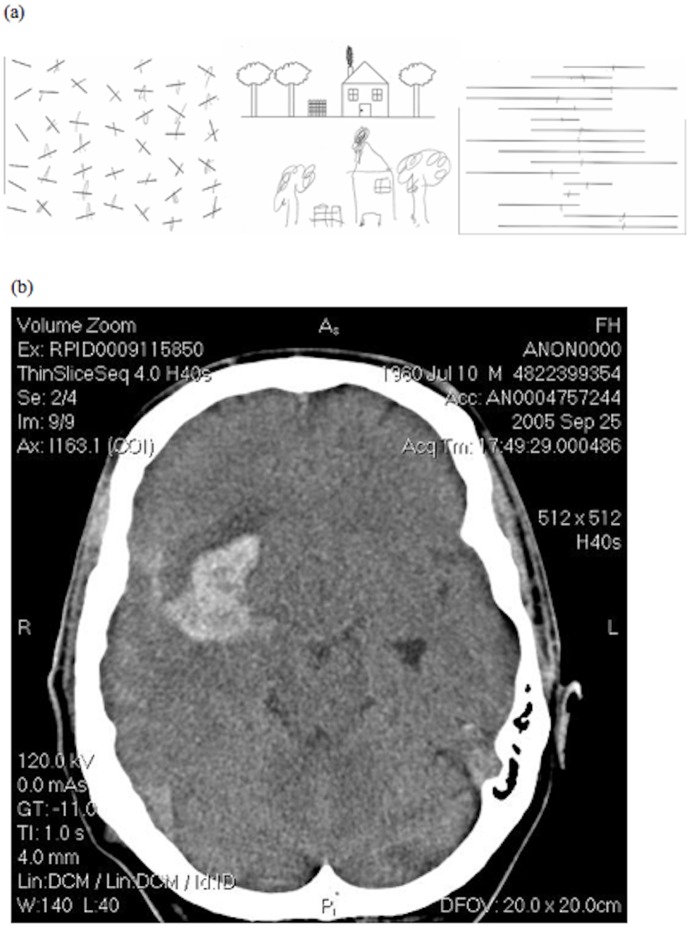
(a) shows some of P1’s screening data for the line bisection, cancellation and scene copying tasks. (b) A CT scan of P1 showing the haematoma with bleeding, and the dark area anterior to it, which is the haemorrhagic stroke.
